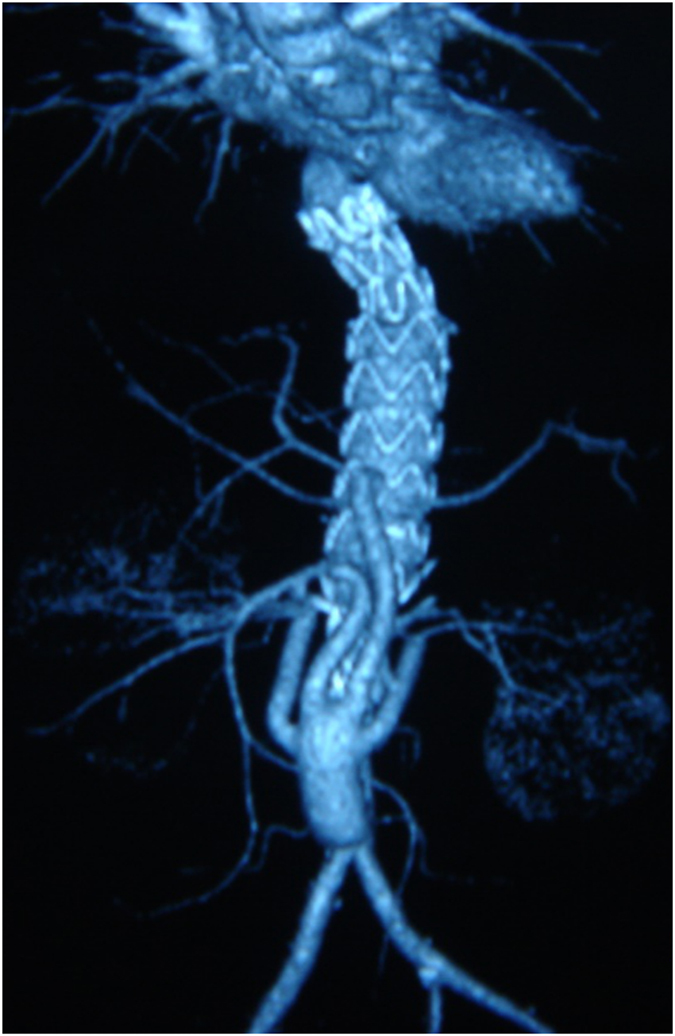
The postoperative CTA imaging of a patient with TAAA, which was underwent the hybrid treatment for TAAA. The quadr-furcated graft was reconstructed from the distal aorta to visceral arteries^27^.
